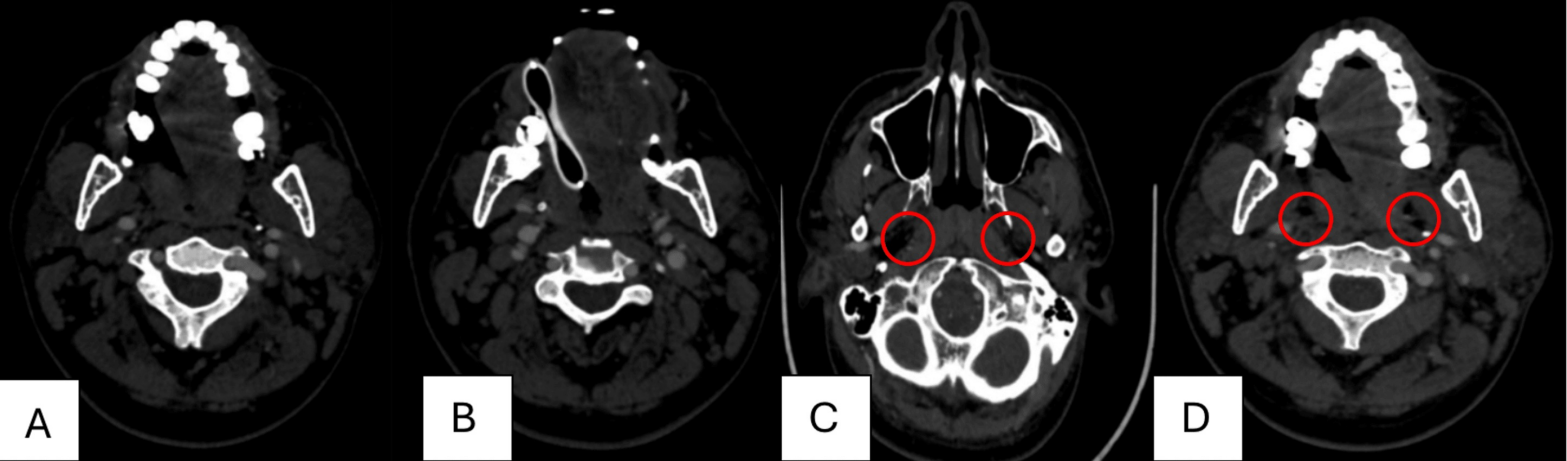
CTA revealed the absence of contrast filling in both ICAs at the level of bifurcation (red circles in C and D) CTA: computed tomographic angiography; ICA: internal carotid artery
